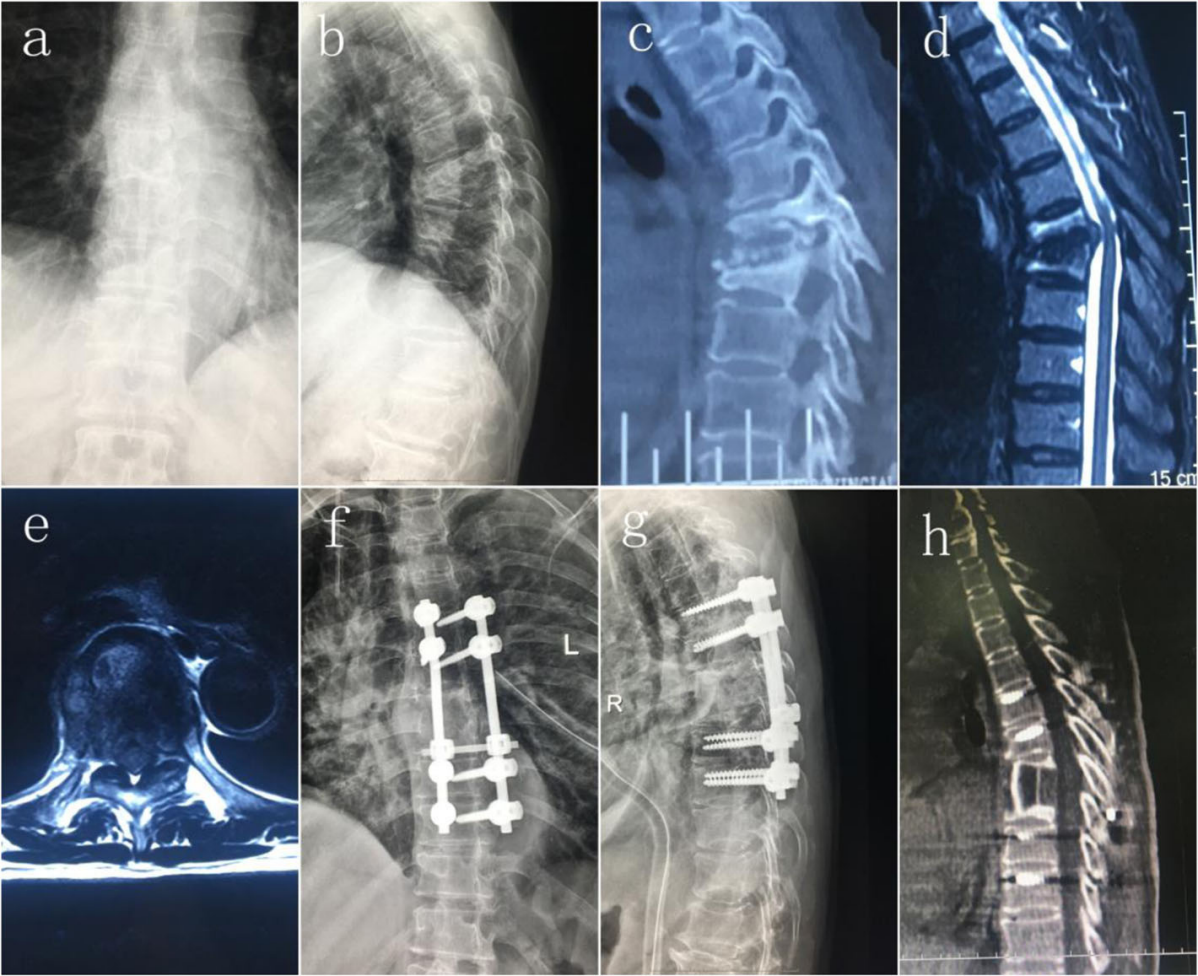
Imaging findings of a 68-year-old patient with thoracic spinal TB who underwent single-stage anterior debridement/bone grafting/posterior instrumentation. a-e Preoperative anteroposterior and lateral x-rays, CT and MR images showing destruction of T6 and T7 and a paravertebral abscess. f-h Postoperative anteroposterior and lateral x-rays and CT showing fixation of T4-T9
